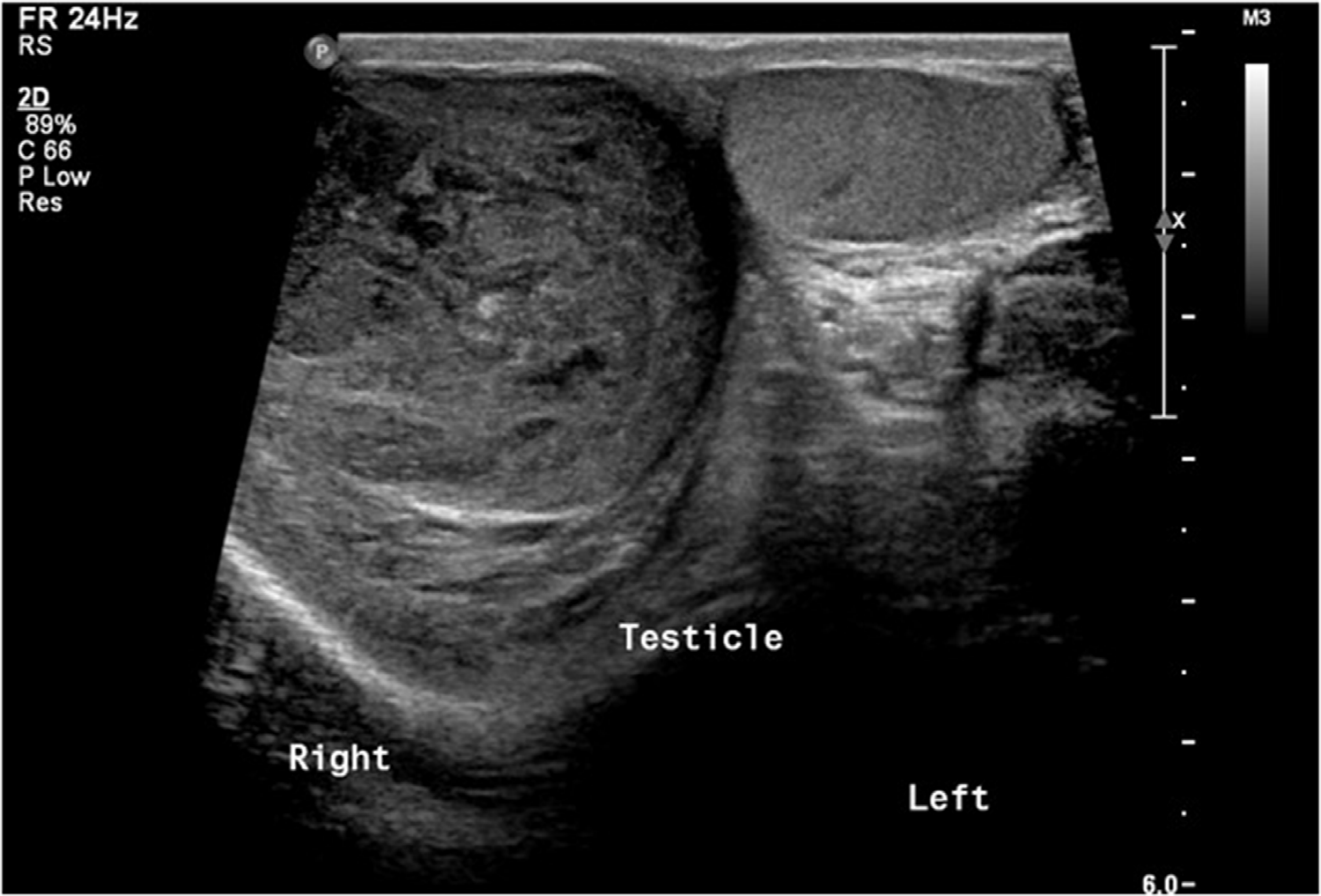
Grayscale ultrasound of the scrotum showed right testicular enlargement secondary to the mass.
